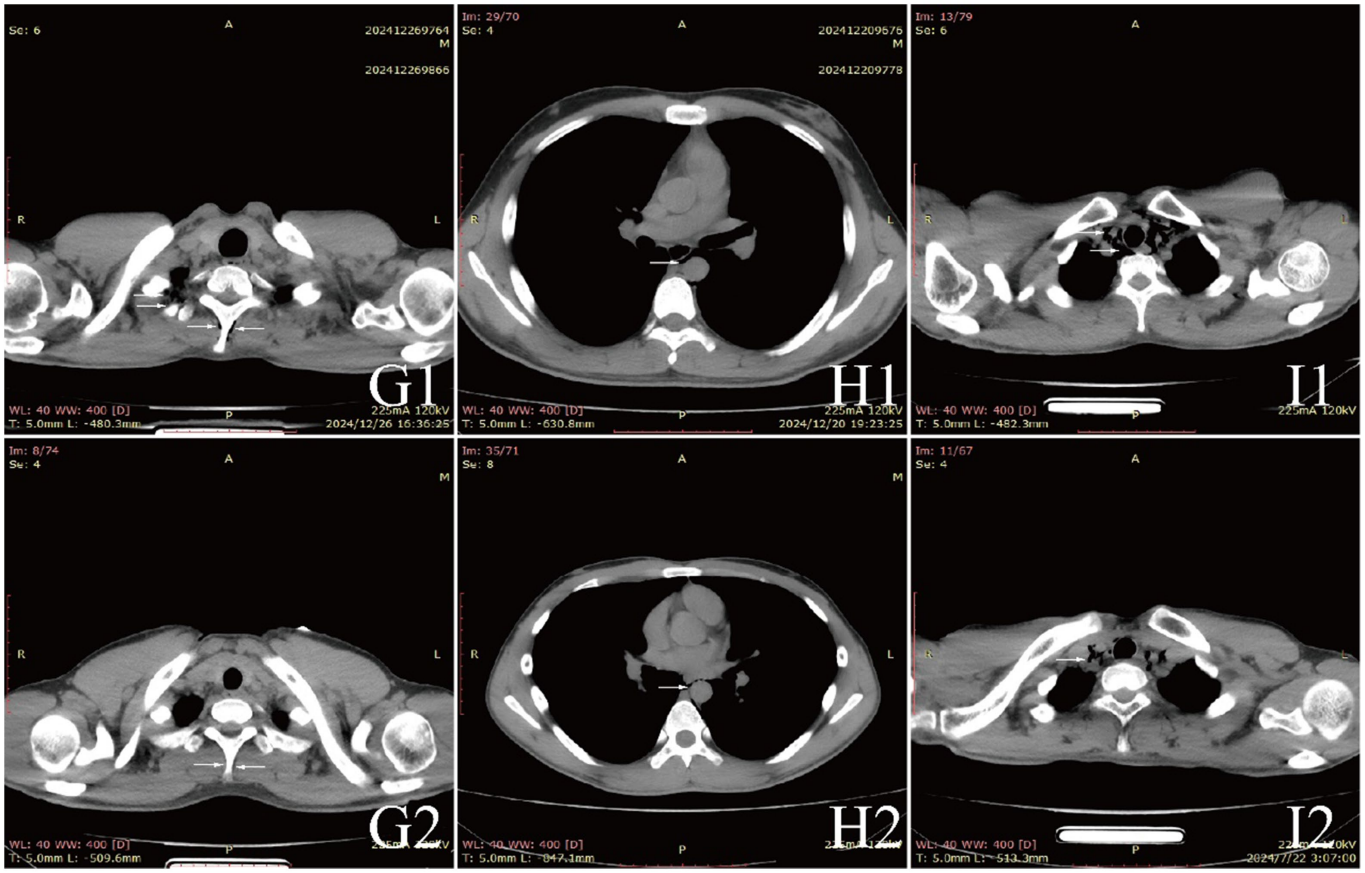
CT comparison images of extra-pulmonary emphysema treatment before and after for Case 7 to Case 9.
